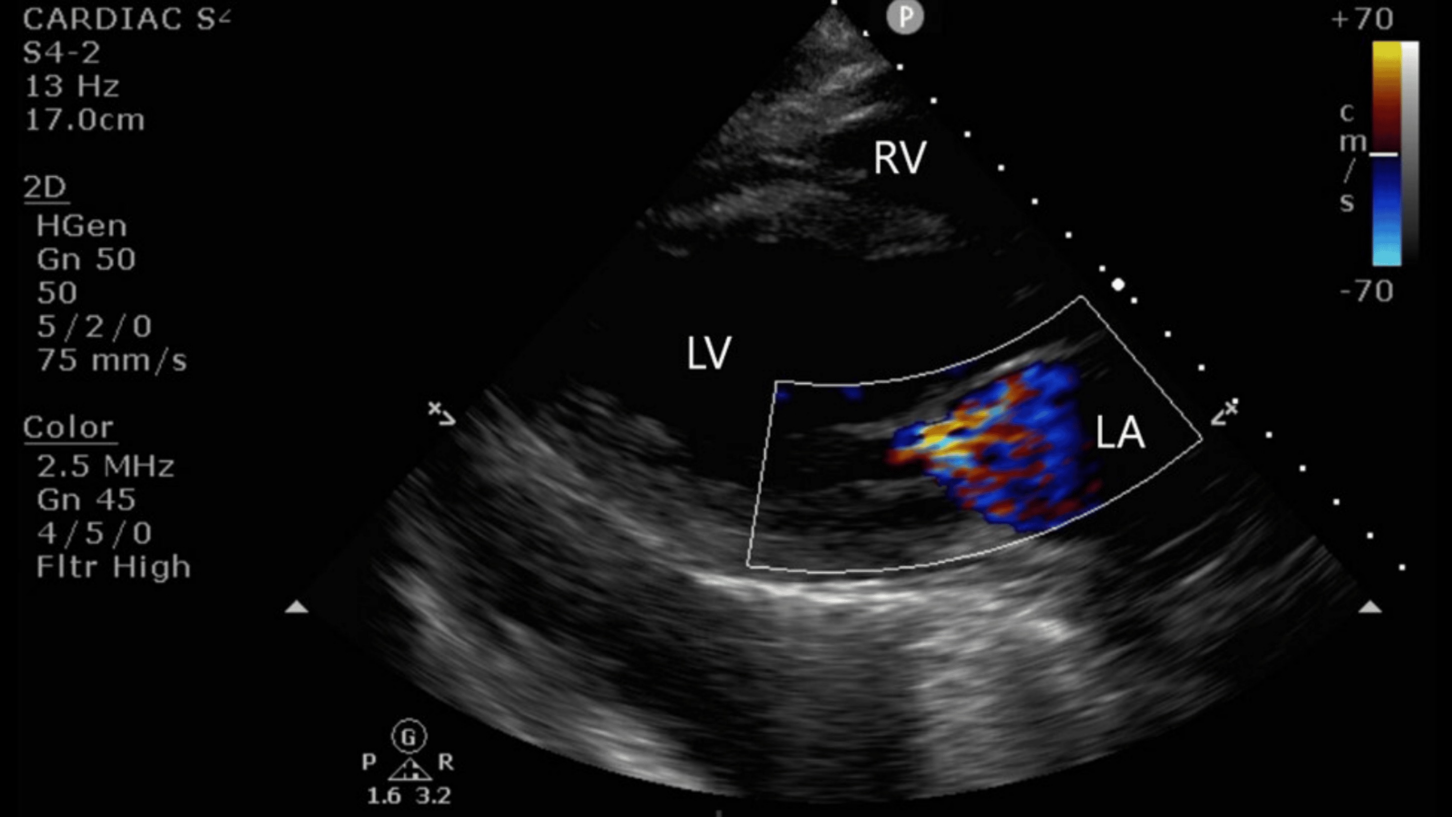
Parastenal long axis The parasternal long axis shows a thickened and dilated left ventricle (LV) and dilated left atrium (LA). Color flow Doppler (CFD) is significant for severe mitral regurgitation (MR). With CFD, severe MR is seen as a large, central jet encompassing more than 40% of the LA. An additional semiquantitative measurement for the advanced POCUS user is the vena contracta measurement. It also utilizes color flow Doppler to measure the narrowest point of the jet as it crosses the valve; greater than 0.7 cm is consistent with severe MR. RV, right ventricle.
